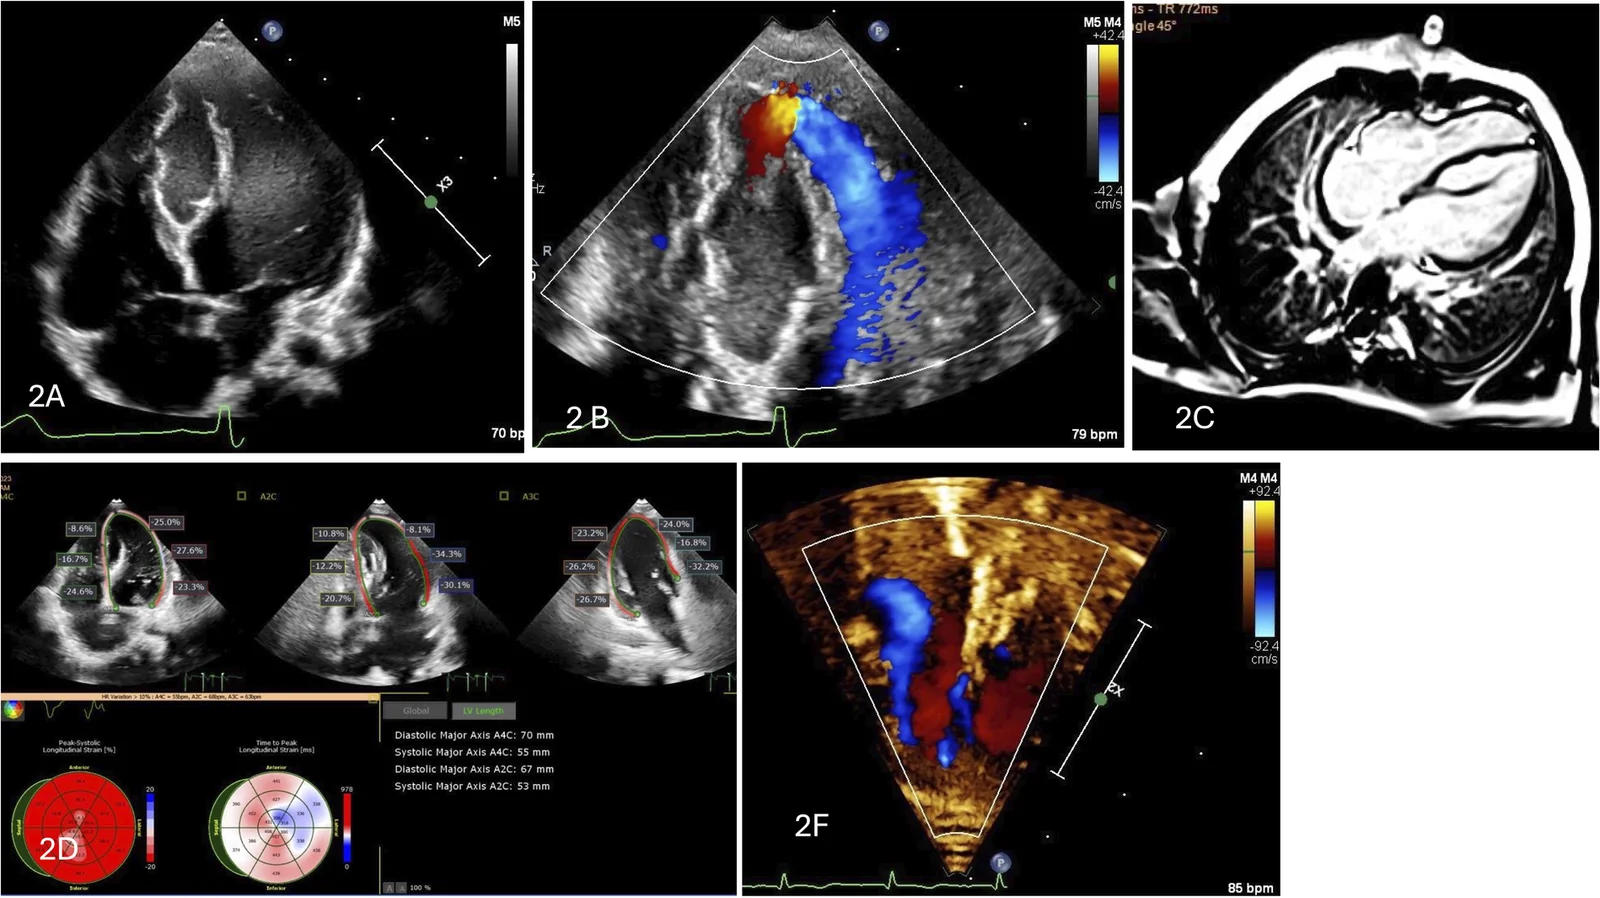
Long-term imaging assessment of a giant interventricular septal hematoma with persistent communication to the left ventricular cavity. (A) Transthoracic echocardiography demonstrating a large chronic dissecting cavity within the interventricular septum. (B) Color Doppler echocardiography showing persistent communication between the hematoma cavity and the left ventricular lumen. (C) Cardiac magnetic resonance imaging (four-chamber view) showing a large blood-filled cavity within the dissected interventricular septum. (D) Segmental strain analysis showing localized impairment of septal myocardial deformation with preserved global ventricular systolic performance. (F) Transthoracic echocardiography showing hyperechogenic structure suggestive of thrombus formation within the apical portion of the dissected interventricular septal hematoma.
